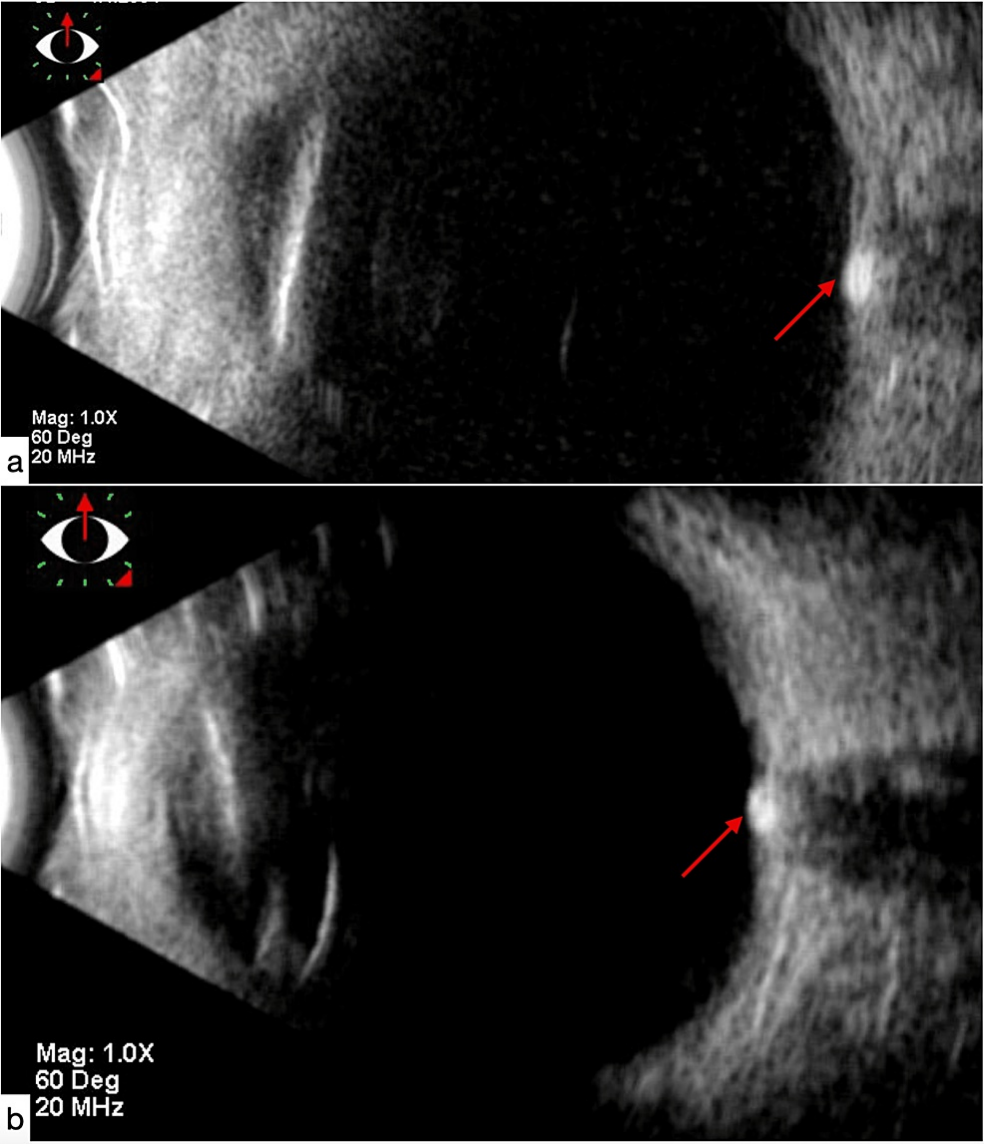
Hyperechogenic appearance of papillary drusen (red arrow) on B-mode ultrasound : (a) right eye, (b) left eye.
